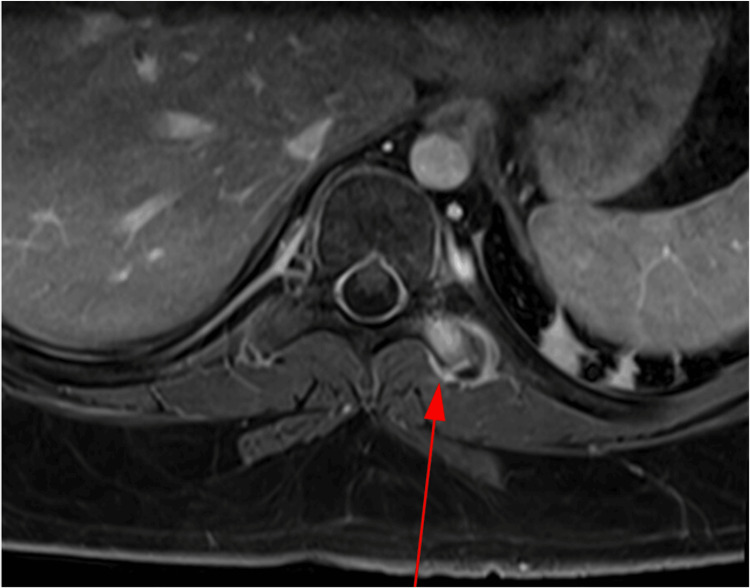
Axial T1-weighted MRI of the thoracic spine The red arrow demonstrates a left transverse process fracture, corroborated on CT of the thoracic spine (not shown).
